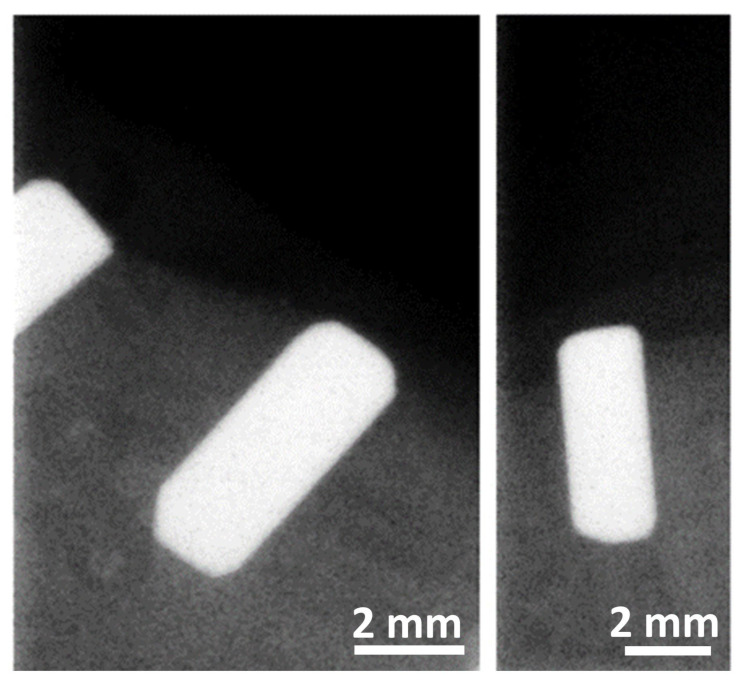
Representative digital radiographs of implanted 3Y-TZP/Ta cylinders six months after surgery.
